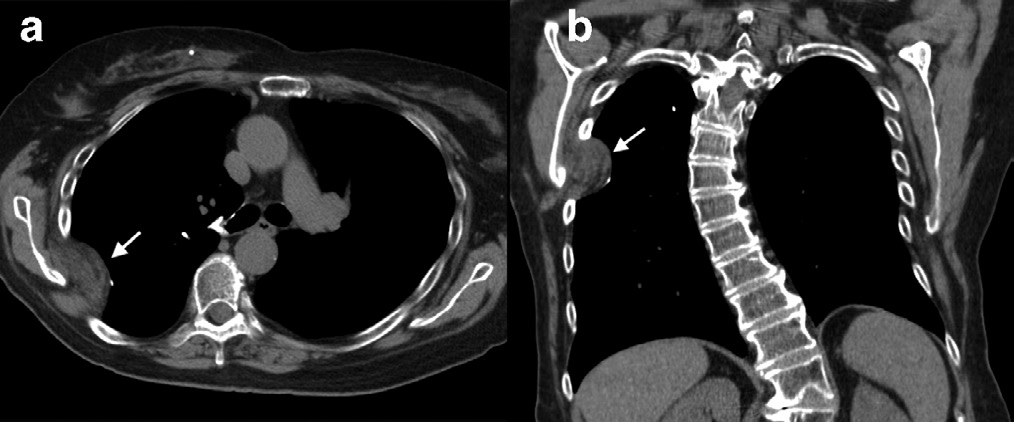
(a, b) Axial and coronal CT images in 79-year-old female (Case 1) showing a unilateral right-sided mixed soft tissue and fatty chest mass herniating medially through a widened rib interspace at a prior thoracotomy site.
